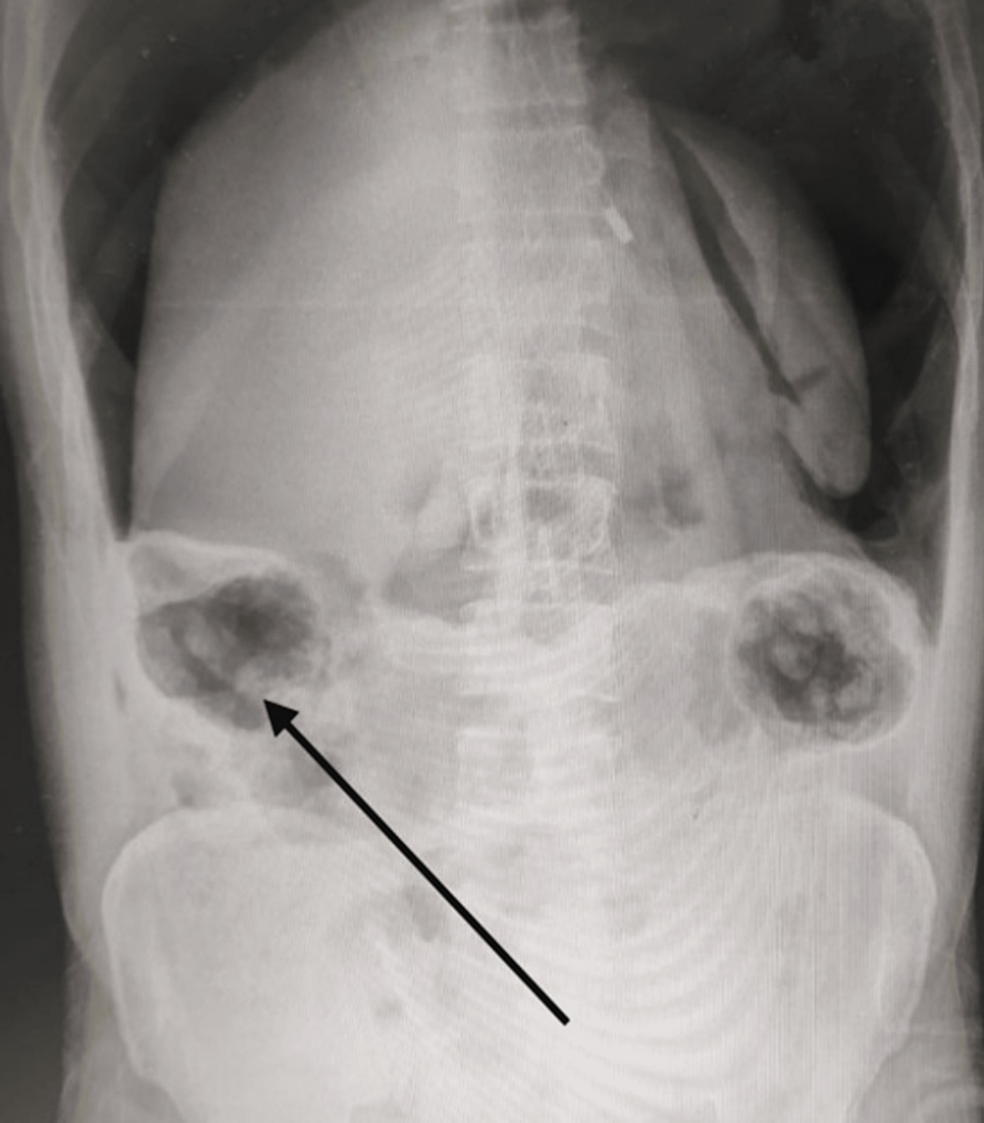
Abdominal X-ray of the patient with gas under the diaphragm suggestive of perforation peritonitis.
